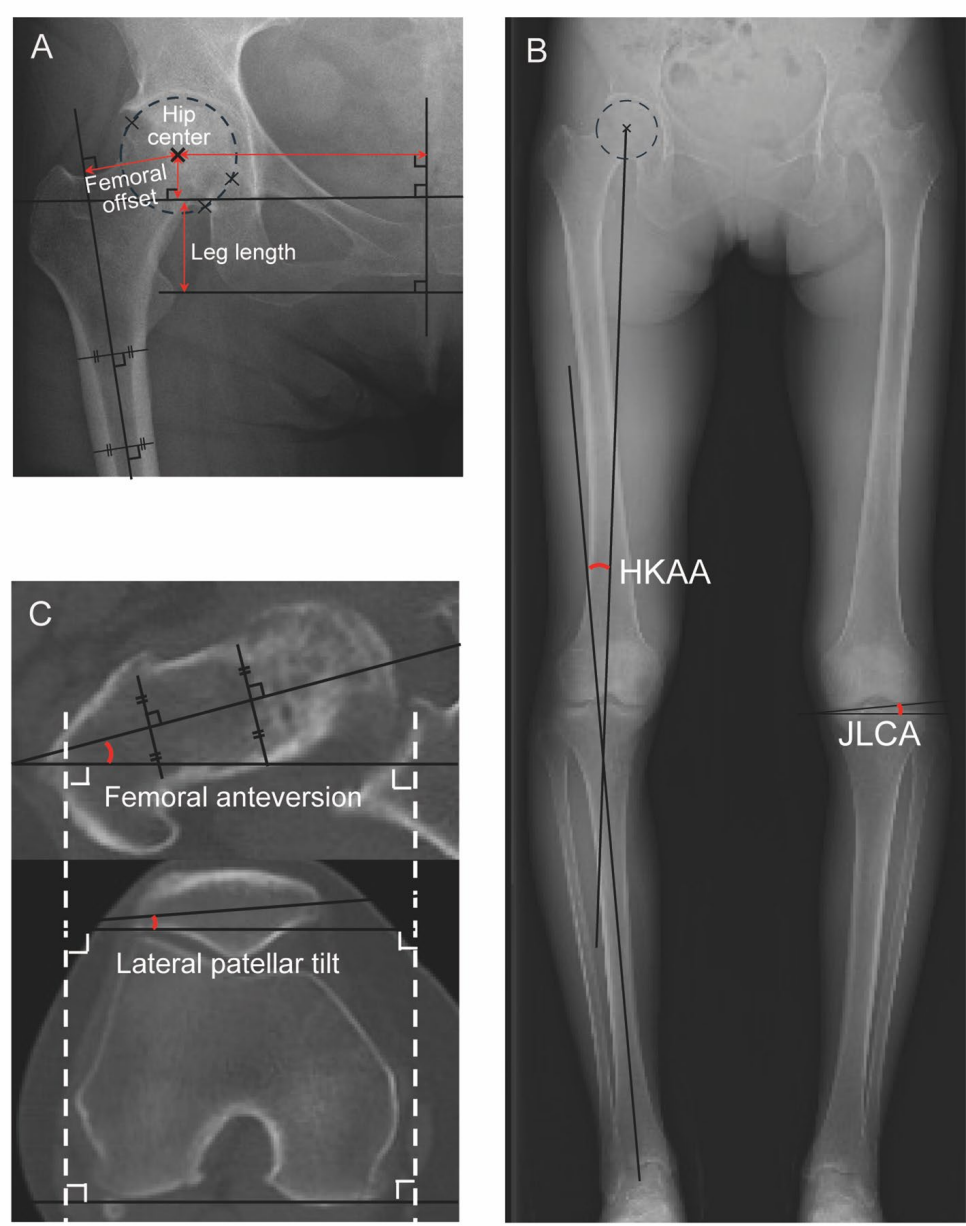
Radiological measurements. (A) Anteroposterior radiograph shows the measurement of hip center, femoral offset, and leg length. The hip center was defined as the center of circle passing through three points set arbitrarily in the intact femoral head. The height and width of hip center were measured as the perpendicular distance from the bilateral teardrops and from the bisector of the teardrops respectively. Femoral offset was measured as the perpendicular distance between the center of the femoral head and the proximal axis of the femur. Apex of the lesser trochanter was used as index of the leg length. (B) Whole-leg radiograph shows the indices used for measuring hip-knee-ankle angle (HKAA) and joint line convergence angle (JLCA). (C) Axial CT image shows the measurement of the femoral anteversion, and lateral patellar tilt based on the posterior femoral condyles.
